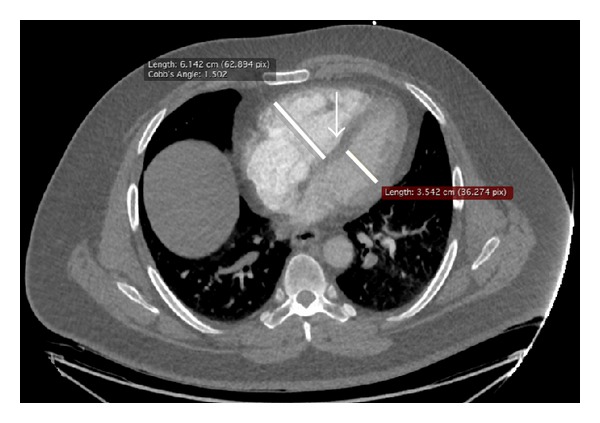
Computed tomography of a 75-year-old man with right-ventricular dysfunction showing increased right-to-left diameter ratio. The short-axis is measured at the widest points between the inner surface of the free wall and the surface of the interventricular septum, in axial transverse images used to diagnose procedure, without reconstructions. The right and left ventricle diameters selected to calculate the ratio are by definition the largest transverse diameters and are therefore often measured in different CTA slices. Note the interventricular septum bowing to the left (arrow).
